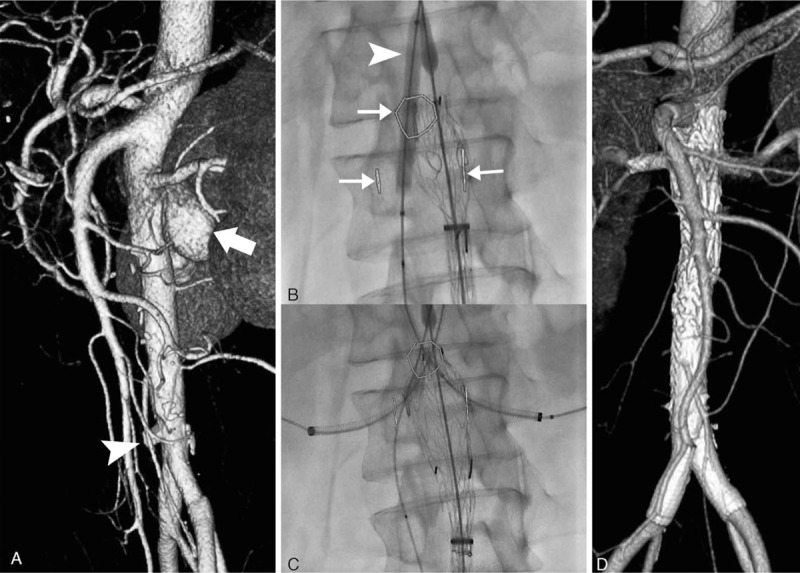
Preoperative CTA (A) showed an abdominal aortic pseudoaneurysm located in the posterior wall of the abdominal aorta at the level of the renal artery (white arrow in A) and an aortic pseudoaneurysm located in the anterior wall of the end of the abdominal aorta (white arrowhead in A). A balloon catheter (EverCross, ev3 Inc.) was intraoperatively (B, C) placed in the superior mesenteric artery (white arrowhead in B) to assist with the release of the stent graft body so that the “V”-shaped scallop could be aligned to the opening of the mesenteric artery. The target arteries’ openings were labeled using image-fusion technology (white arrows in B) so that they were always visible in fluoroscopy imaging. Using the approach from the upper-limb arteries, the bilateral renal arteries could be selected through the fenestrations (C). The CTA follow-up at 6 months after surgery showed that (D) the circulation of the superior mesenteric artery and bilateral renal arteries was smooth and that the pseudoaneurysm was isolated completely, with no endoleak. CTA = computed tomographic angiography.
